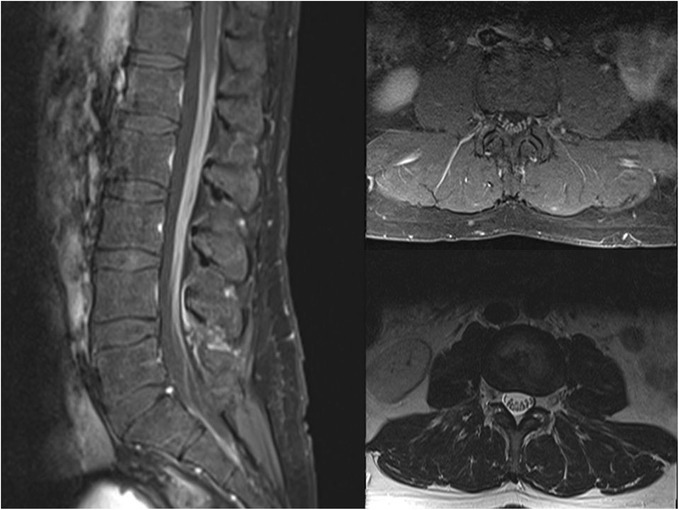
Initial MRI lumbar spine following Ipilimumab. Sagittal and axial T1w fat (left and top right) saturated post contrast and axial T2w FSE (bottom right) images demonstrating smooth, non-nodular avid enhancement of cauda equina without significant nerve root thickening
